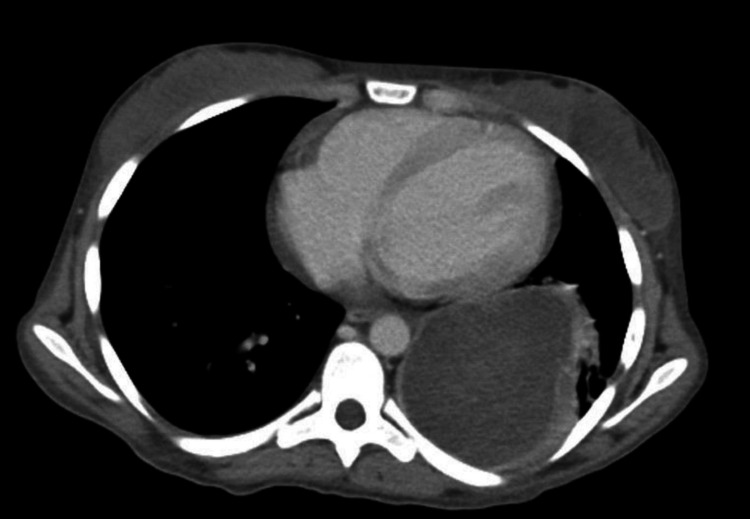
Axial enhanced CT scan showing a cystic lesion with atelectasis in the left lung.
